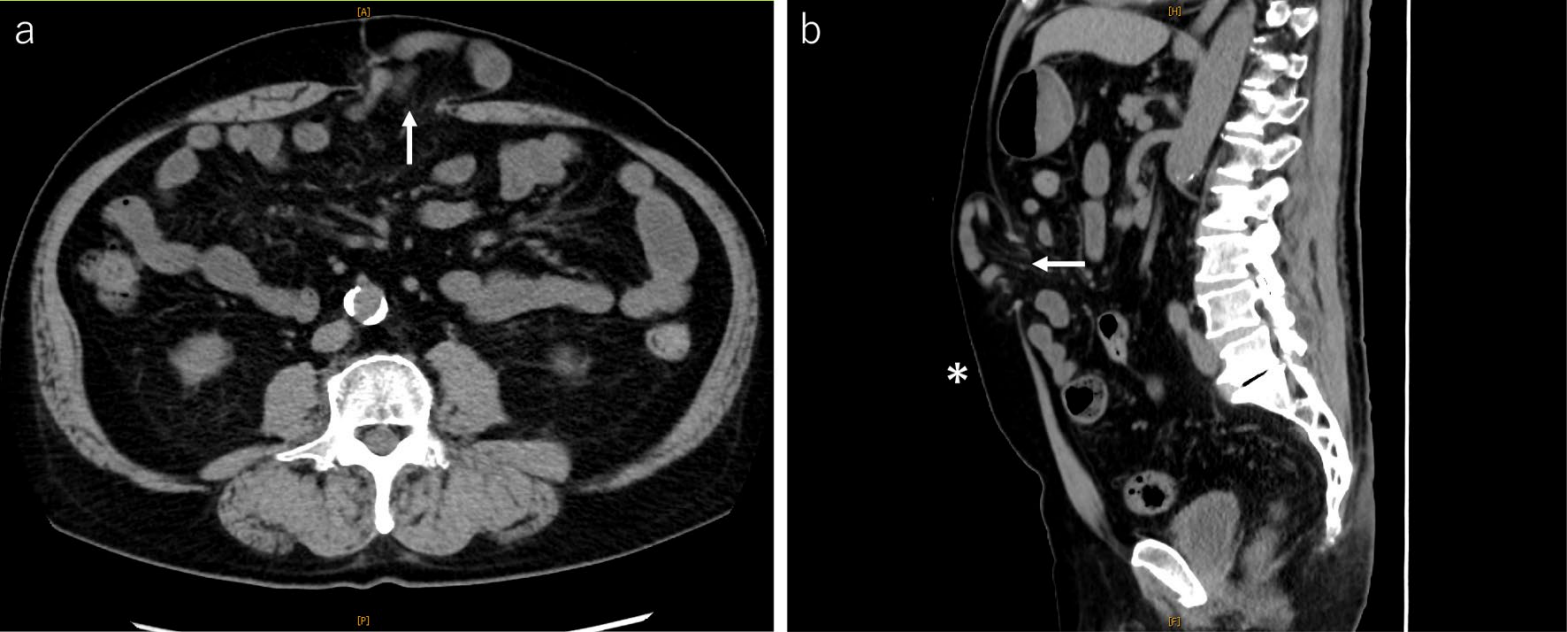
Abdominal computed tomography. Abdominal computed tomography demonstrates an incisional hernia orifice in the upper abdomen. a Axial image showing the hernia defect measuring 3.7 cm wide (arrow). b Sagittal image showing the hernia defect measuring 4.0 cm long (arrow). The defect is located in the upper umbilicus (*)
